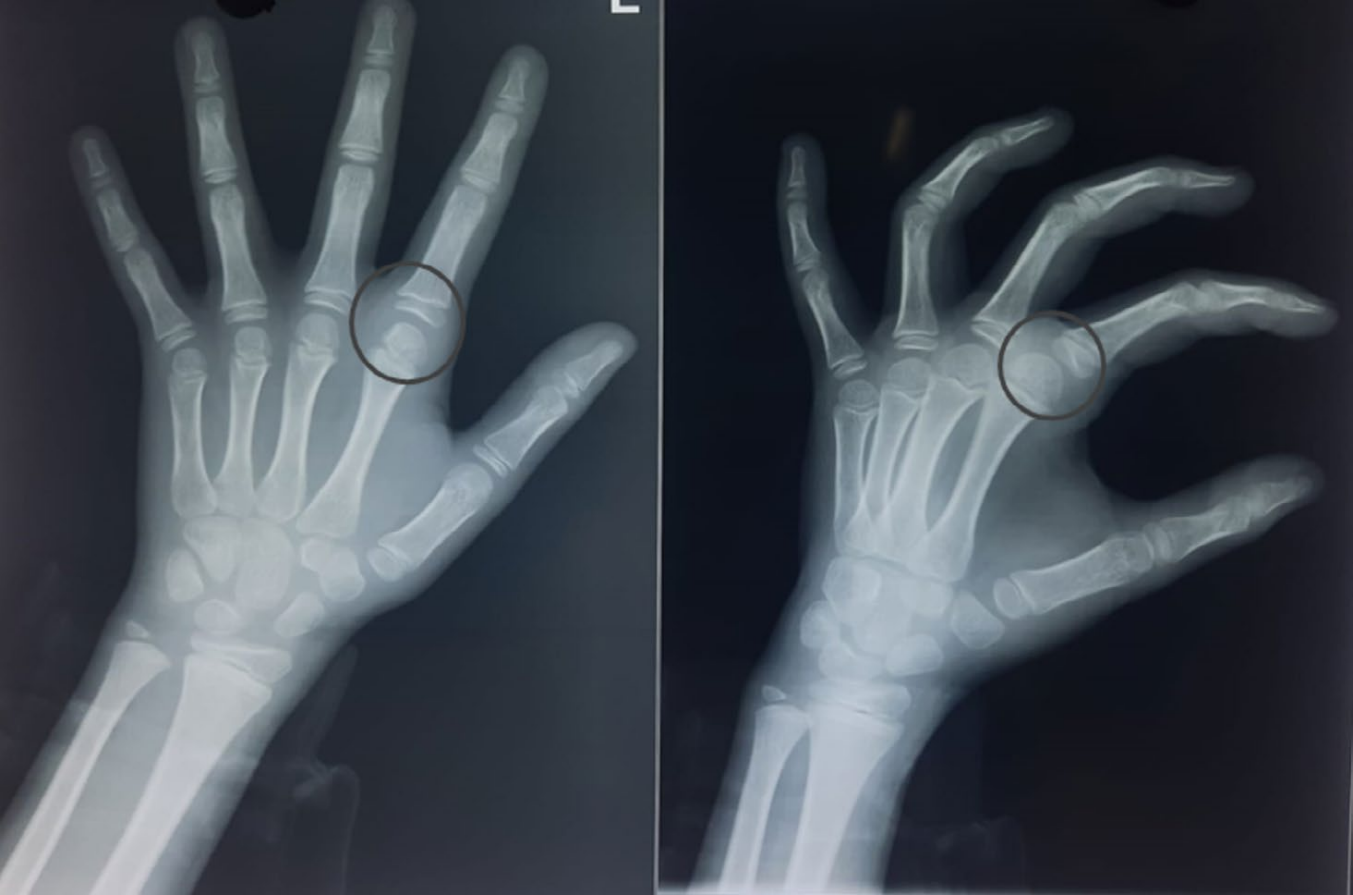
Follow up radiographs at 6 months showing well‐reduced left index finger MCP joint (rings).
